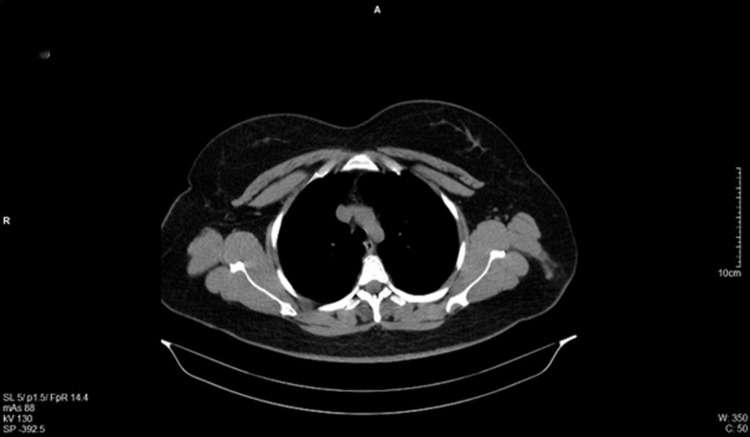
Normal axial CT scan, highlighting a section with no evidence of active disease of distant metastases (no detectable masses, lymphadenopathy, or organomegaly)
